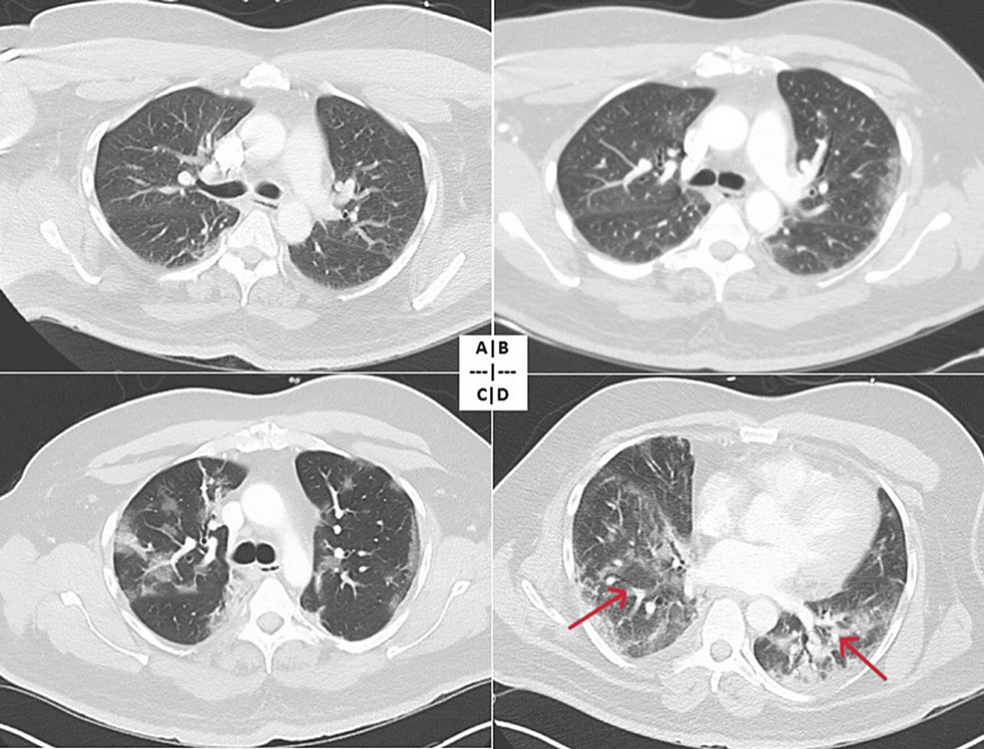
CT scan of the chest showing the development of secondary organizing pneumonia (patient 1). (A) CT of the chest on initial presentation with minimal ground glass opacities. (B) CT of the chest at two weeks showing interval development of bilateral ground glass opacities. (C) CT of the chest at three weeks with worsening bilateral ground glass opacities. (D) CT of the chest at four weeks with extensive bilateral consolidation (red arrows). CT: computed tomography
